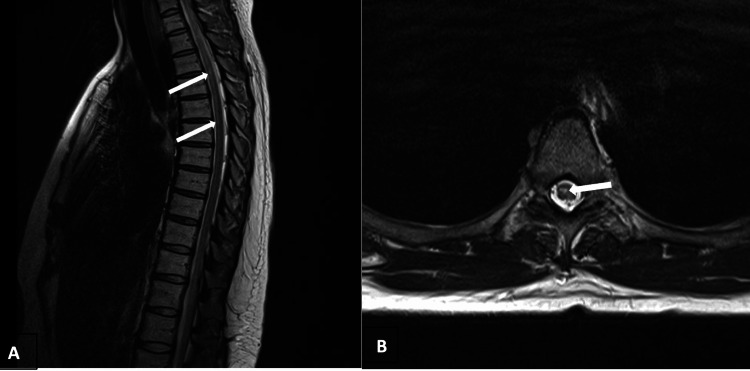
Sagittal (A) and axial T2-weighted MRI of the thoracic spine showing linear T2 signal hyperintensity in the right paracentral spinal cord extending from T2 through T5.
